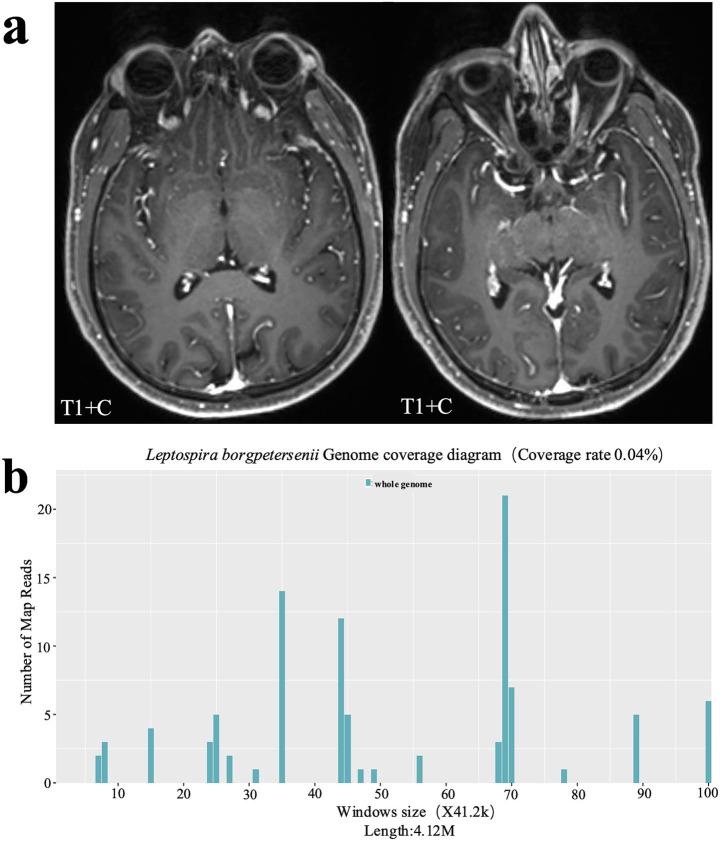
Imaging findings and sequencing result of the patient. (a) Magnetic resonance imaging (MRI) of the brain revealed mild dural and leptomeningeal enhancement. T1 + C, contrast-enhanced T1-weighted imaging. (b) MetaCAP indicated 34 sequence reads of *Leptospira borgpetersenii* were identified with a coverage rate of 0.04%, which supported the diagnosis of a *Leptospira borgpetersenii* infection manifesting as aseptic meningitis.
